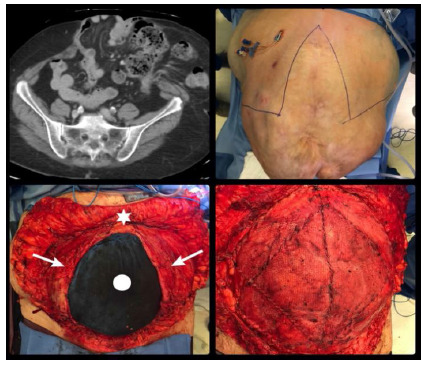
CT scan (top left) demonstrating a multicompartment large ventral hernia with loss of domain. Fleur de Lis incision (Top Right), Note the Right upper quadrant catheter utilized for preoperative PPP after BTA Injection. Completed dissection of the hernia sac (bottom left) with exposure of a 23x20cm defect (fascial edges marked by white arrows). The patient’s head is oriented superiorly (white star) while the abdominal contents are covered with a blue towel (white circle) - polypropylene onlay mesh reinforcement (bottom right) after primary fascial closure. Myofascial release was not necessary due to the effect of BTA and PPP.
